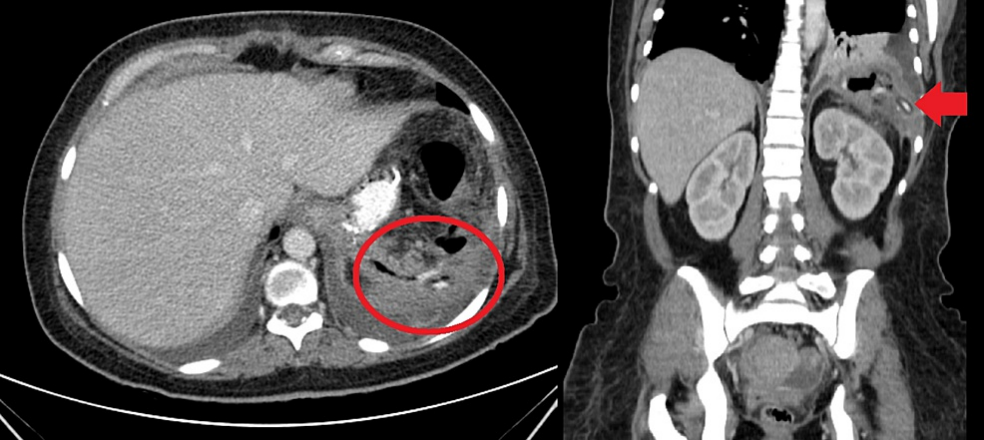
A postoperative (fourth day) CT scan showing contrast inside the drain, a sign of gastric leak The postoperative abscess is marked with a circle, and the contrast inside the drain is marked with an arrow.
